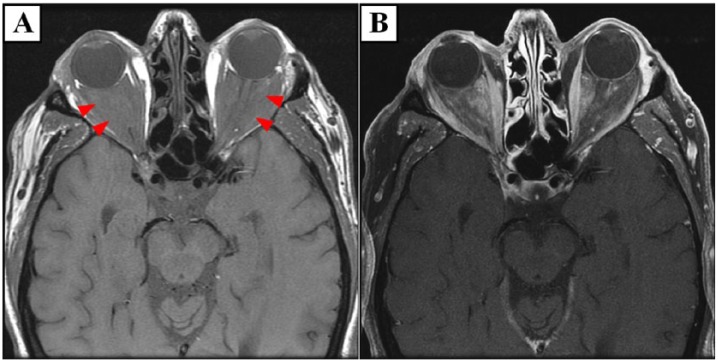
Head magnetic resonance imaging. T1-weighted head magnetic resonance images revealed intraconal abnormal soft tissue which completely fills the orbits bilaterally (A) and enhances after gadolinium administration (B).
